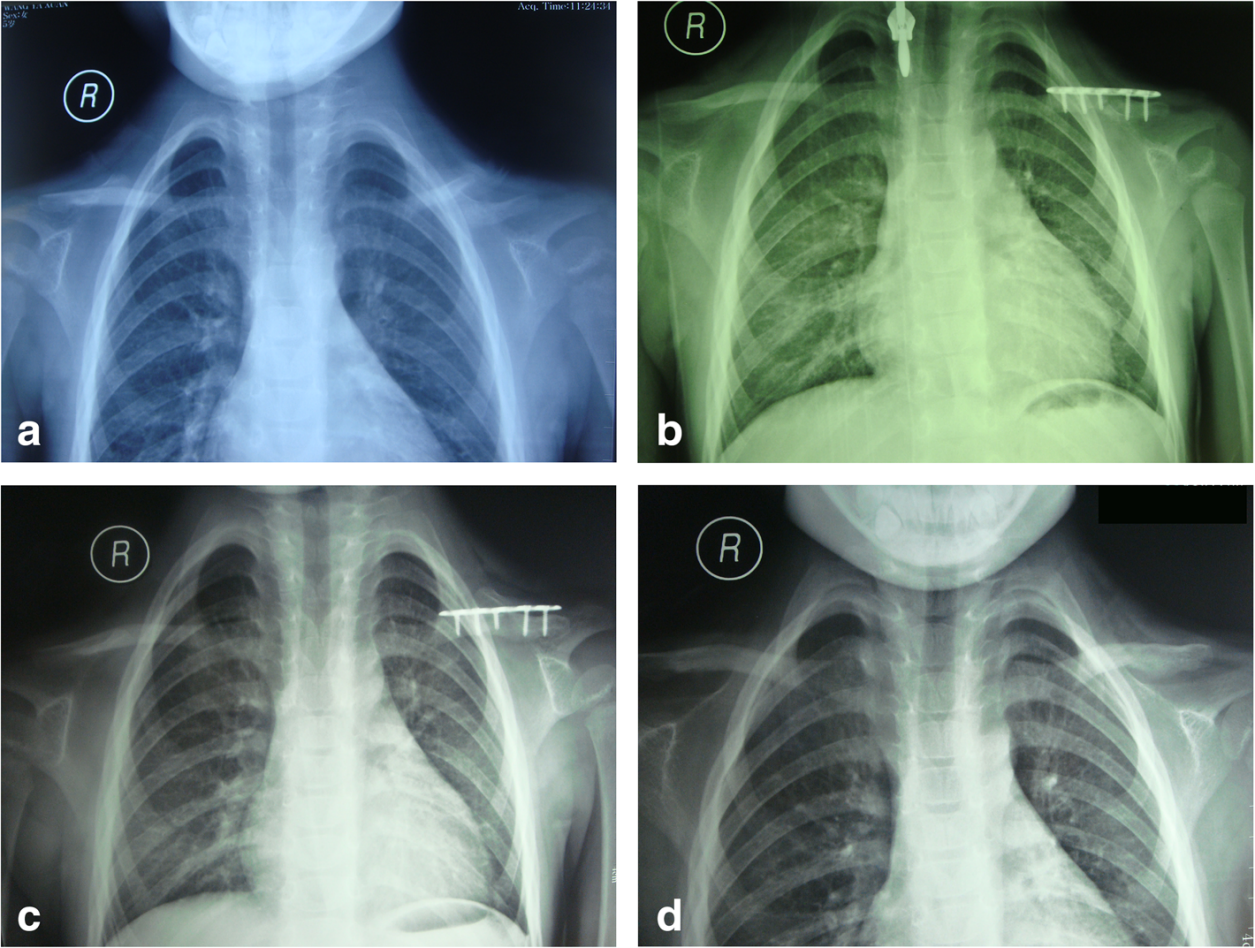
a Radiographic presentation of a 4.9-year-old girl with left clavicular pseudarthrosis preoperatively. b Postoperative radiograph with pseudarthrosis resection and iliac crest bone autograft stabilized with plate. c 11 months postoperative follow-up. d 8 months after removing the plate
